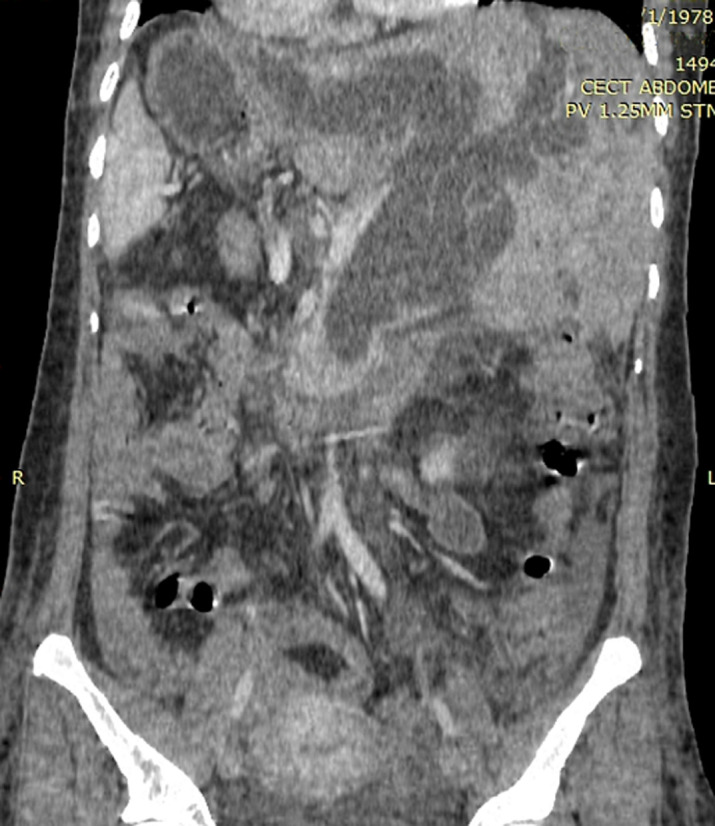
computed tomography revealed a fusiform dilatation of the extrahepatic and intrahepatic ducts with the CBD
